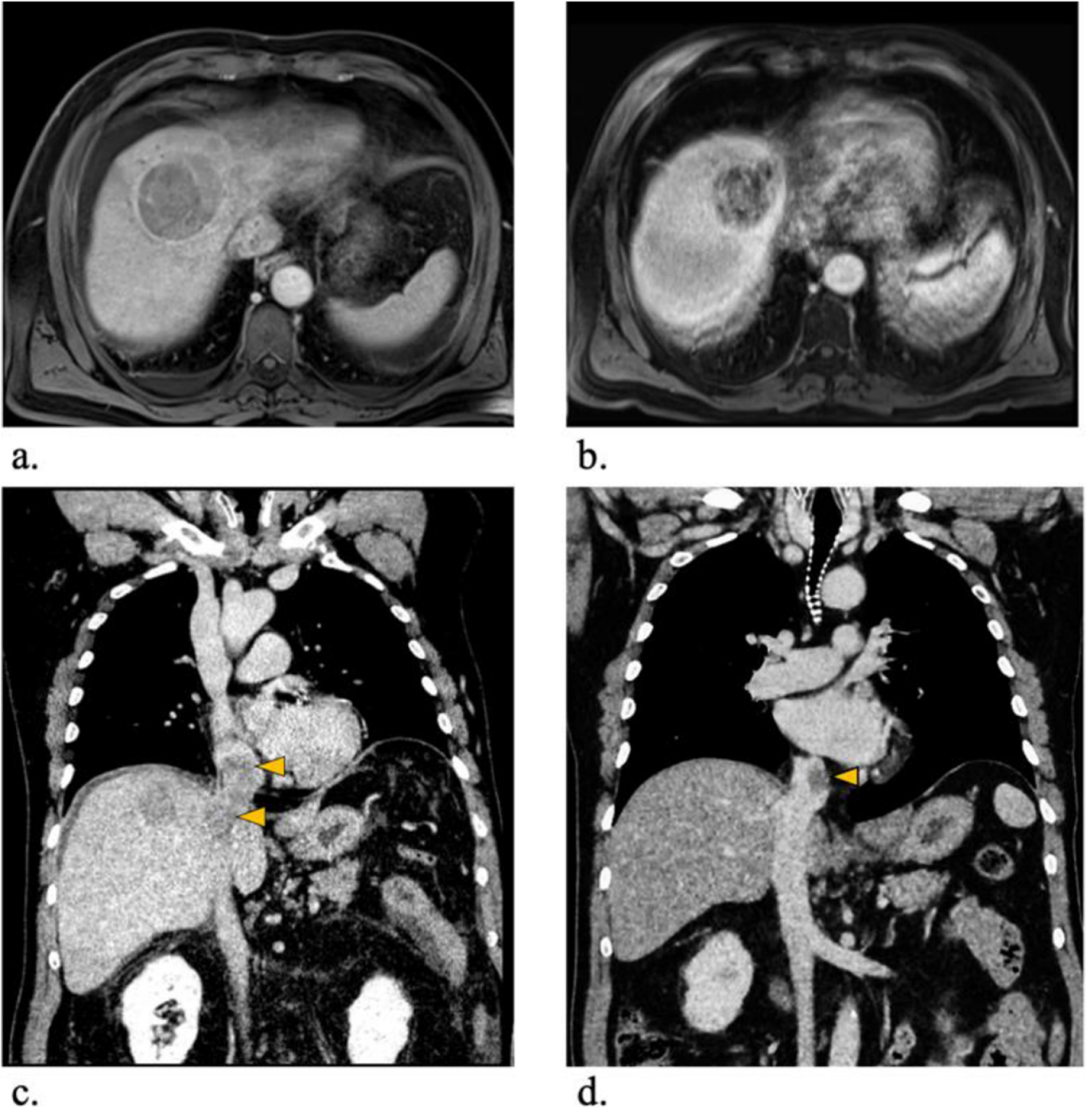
Pre- and post-interventional radiographic images. (A) Pre-interventional contrast enhanced MRI examination in the hepatobiliary excretion phase showing a large tumor lesion in segment IV/VIII in transversal plane. (B) six-months post-interventional MRI showing good radiological response to treatment using the same imaging technique. (C) Pre-interventional contrast enhanced CT examination confirms the presence of a TT entering the middle liver vein and extending through the IVC into the RA of the heart in the coronal plane. (D) six-months post-interventional CT showing marked shrinkage of the TT improving blood flow to the heart using the same imaging technique
